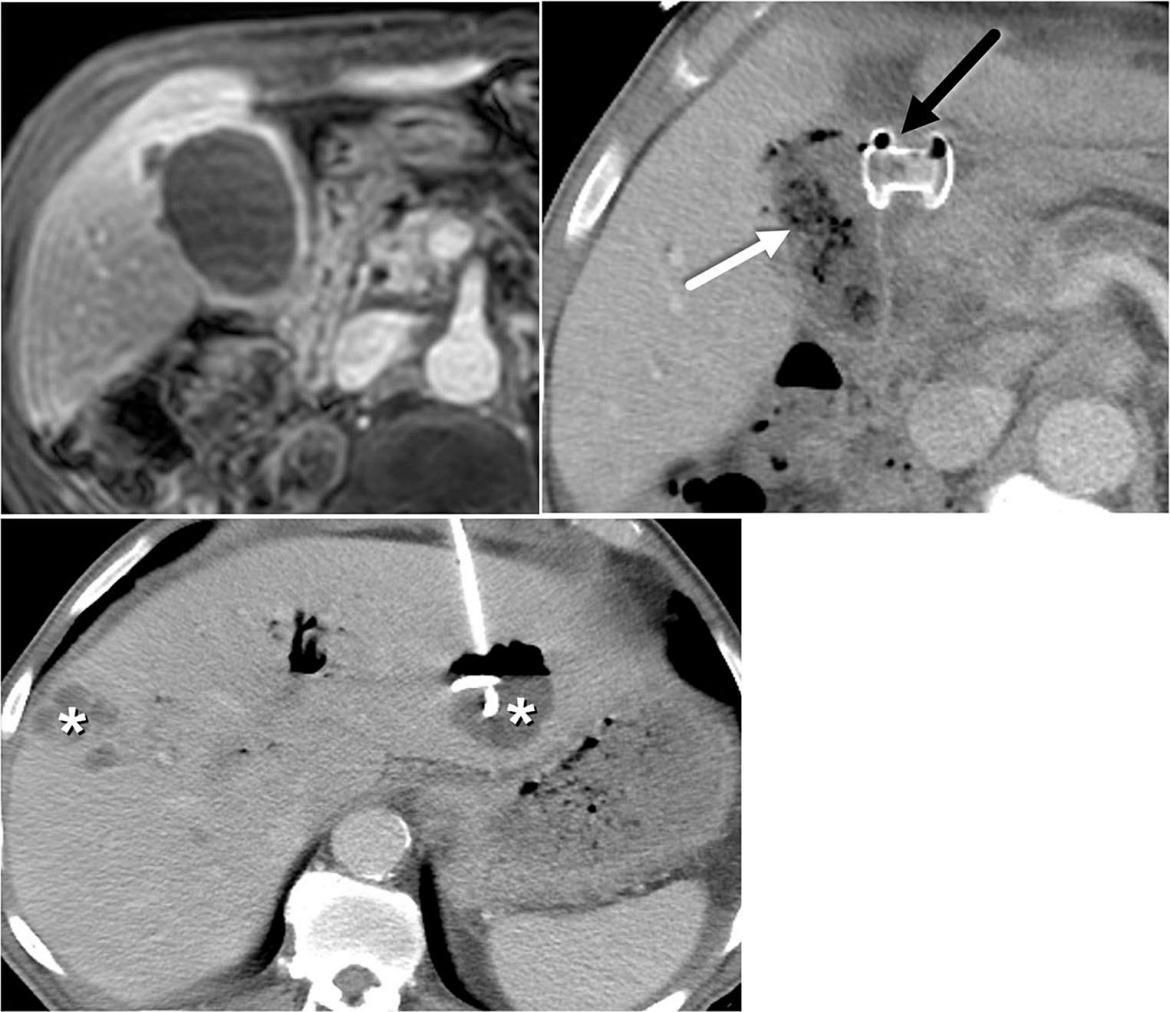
72 year-old female with pancreatic neck cancer and acute cholecystitis. A. Post-contrast axial LAVA MRI shows irregular gallbladder wall with focal outpouchings indicating contained gallbladder wall perforation (white arrows) related to gangrenous cholecystitis, a contraindication to endoscopic gallbladder drainage, which was not recognized prospectively. B. LAMS cholecystogastrostomy was subsequently placed (black arrow). Expected air in the partly decompressed gallbladder is noted (white arrow). C. The patient developed multiple hepatic abscesses (white asterisks) requiring percutaneous drainage, and ultimately succumbed to sepsis
